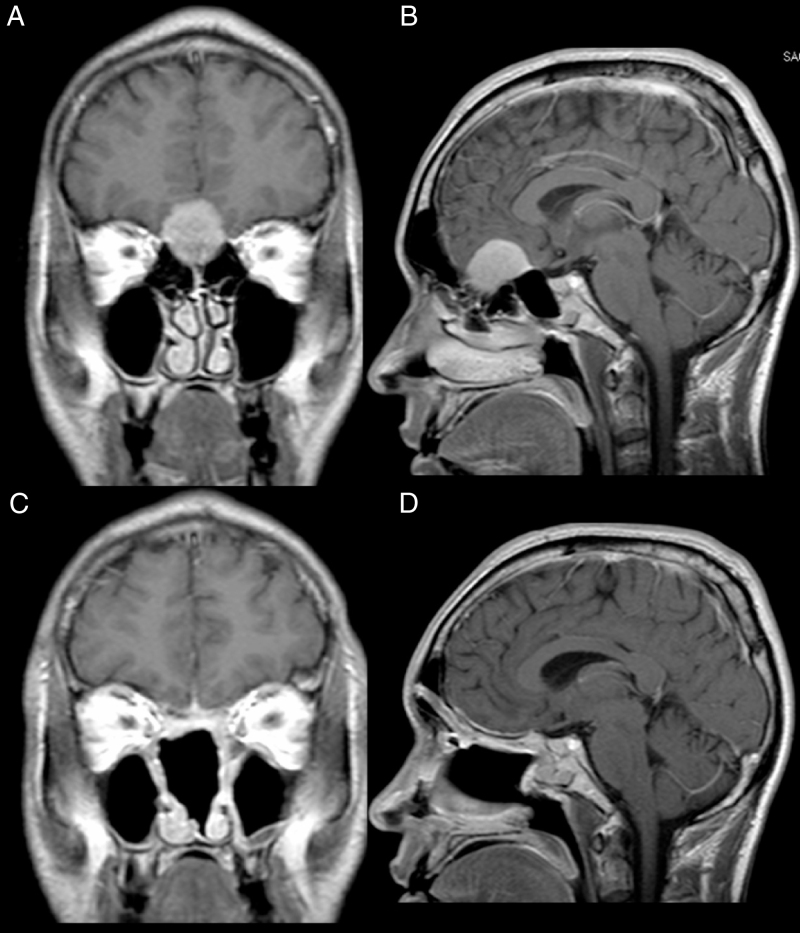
Patient 2, male, 39 years old. (A, B) Preoperative MRI with contrast showing an intracranial mass with dural attachment and invasion of the anterior and posterior ethmoid. (C, D) Postoperative 1 year MRI with contrast, showing gross total resection and no signs of tumour recurrence or brain herniation. Both flaps can be seen with gadolinium enhancement and preservation of the pedicles and viability of the endoscopic double flap.
